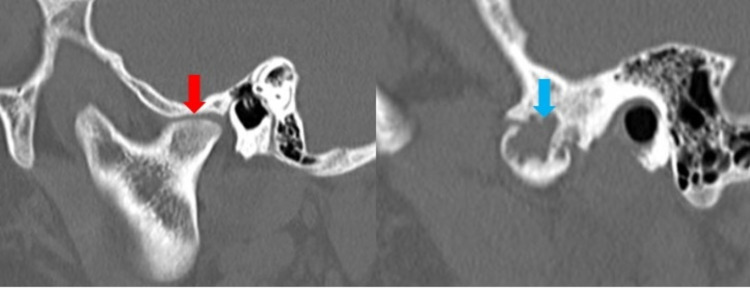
(A) CT image of medial articulation of BMC of the left TMJ in sagittal view; (B) CT image of lateral articulation of BMC of the left TMJ in sagittal view. a) Flattened articulation shown (red arrow) b) erosive joint changes (blue arrow). BMC: Bifid mandibular condyle; TMJ: Temporomandibular joint.
